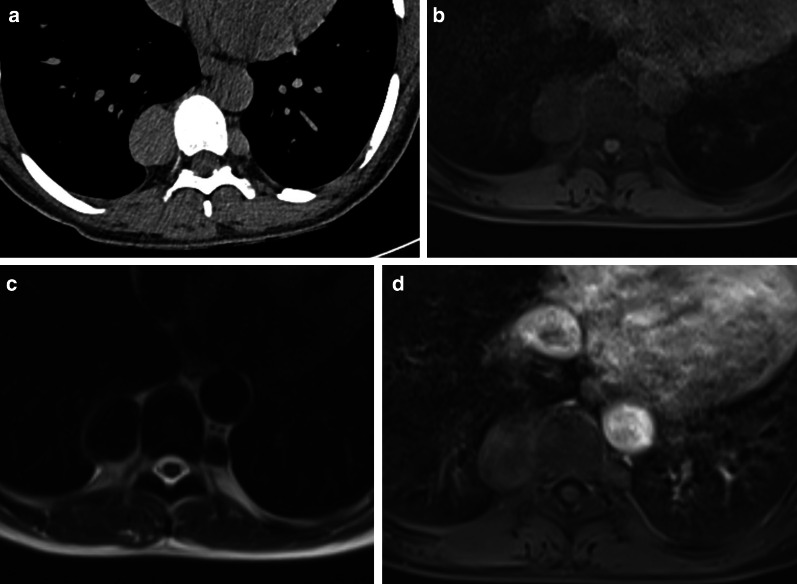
Diagnosis: extramedullary hematopoiesis. Technique: chest CT and MRI. Description: A 42-year-old woman with sickle cell anemia was referred for a routine control examination. The axial CT in the mediastinal window setting (a) shows as incidental findings, two well-delineated paravertebral masses with a homogeneous hypodense aspect. There is no locoregional bone invasion. On both the axial T1 vibe MRI sequence with FS (b) and the axial T2 haste MRI sequence (c), the lesions have the same signal intensity as bone marrow. There is no contrast enhancement of the lesions on the axial T1 vibe sequence with FS (d)
